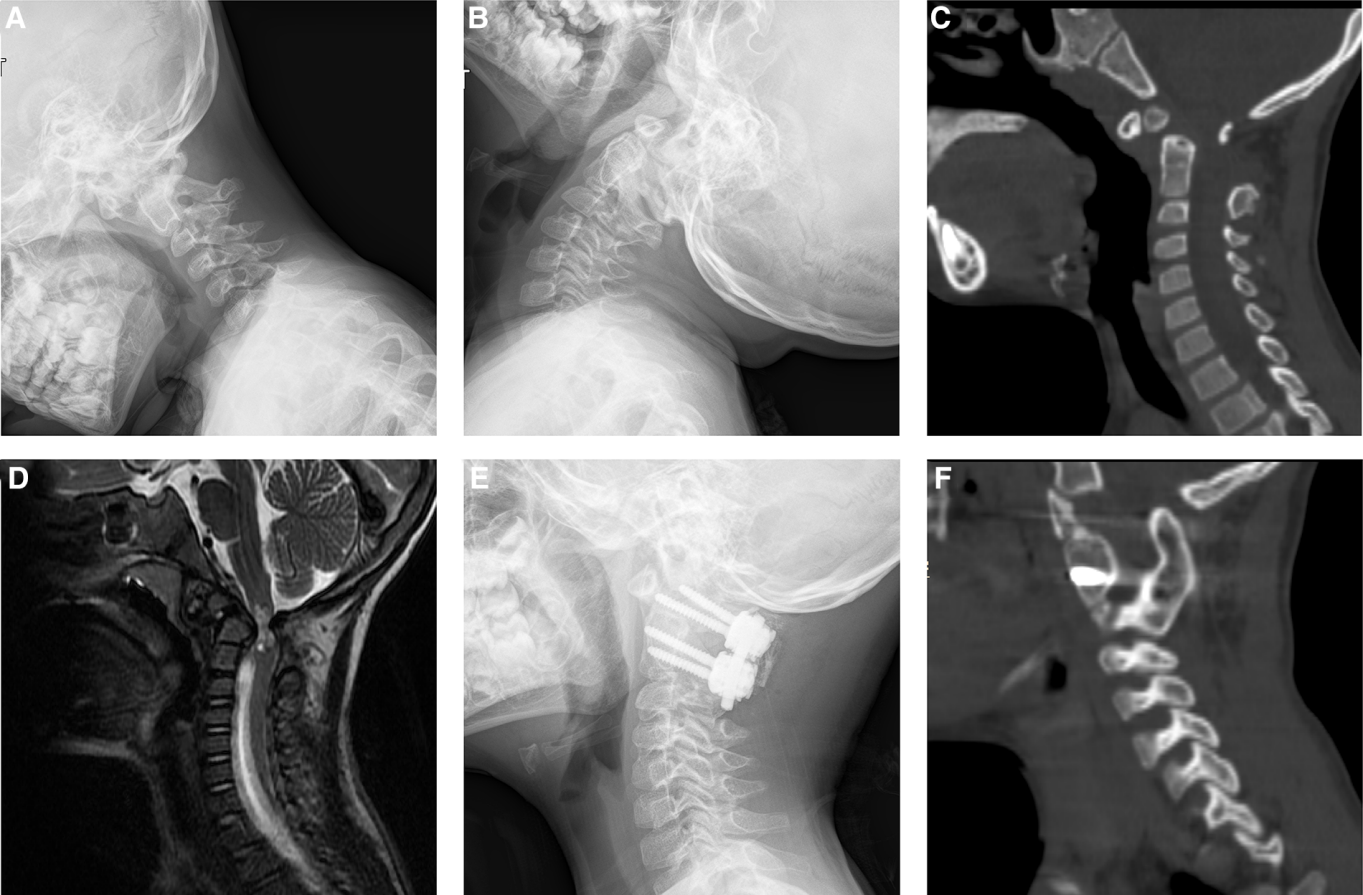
Images of a 5-year-old boy diagnosed of os odontoideum and underwent atlantoaxial fusion with the use of IBG. Preoperative plain radiograph of flexion (A) extension (B) showed atlantoaxial dislocation. Preoperative CT showed os odontoideum (C). Preoperative magnetic resonance imaging (MRI) showed spinal cord compression (D). Postoperative plain radiograph (E) showed the rigid screw/rod fixation. CT sagittal reconstruction showed solid bony fusion 6 months after surgery (F).
